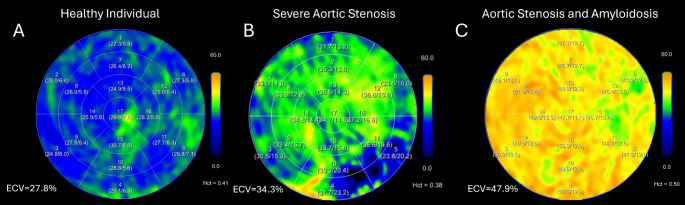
Bullseye Plots of Global CT-ECV Values in Different Cardiac Disease States. Bullseye plots of 17-segment models of left ventricles in (A) a normal individual with a mean CT-ECV of 27.8%, (B) an individual with severe aortic stenosis and a mean CT-ECV of 34.3% and (C) an individual with combined severe aortic stenosis and amyloidosis with a mean CT-ECV of 47
